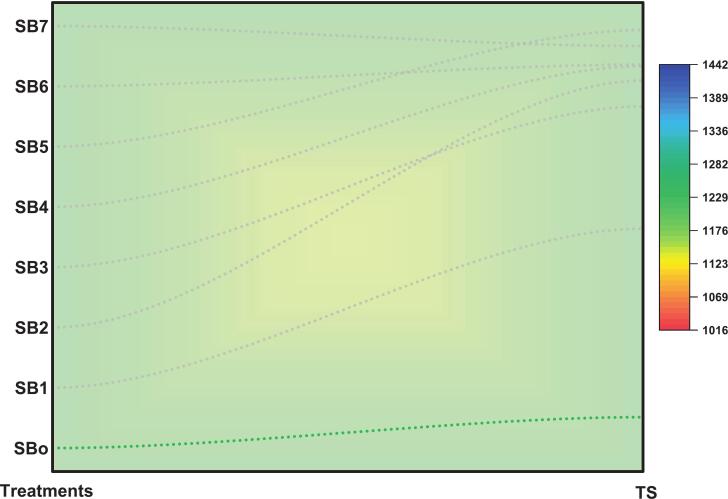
Total sugar content (mg GE/mL) of sea buckthorn juice samples (SBo–SB7) after ultrasound-assisted fermentation. SB5 exhibited the highest sugar concentration, indicating enhanced saccharification and carbohydrate release through combined microbial and ultrasonic treatment, n = 3 replicates.
